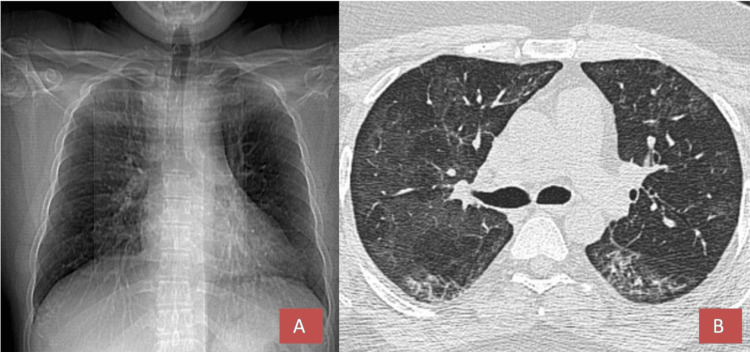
High-resolution computed tomography findings The HRCT scout image of the chest (A) in axial view (B) at the four-week follow-up indicates a reduction in pneumonitis and pulmonary edema.
